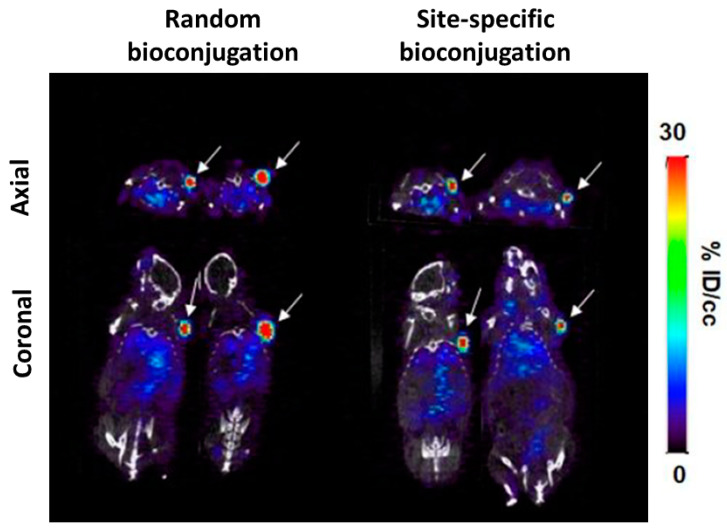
Single slice PET/CT images of random (left) and site-specific unnatural amino acid pAMF-DBCO SPAAC (right) conjugated [^89^Zr]Zr-DFO-Trastuzumab 96h post injection. Adapted with permission from Ahn et al. [53]. Copyright 2020, American Chemical Society.
